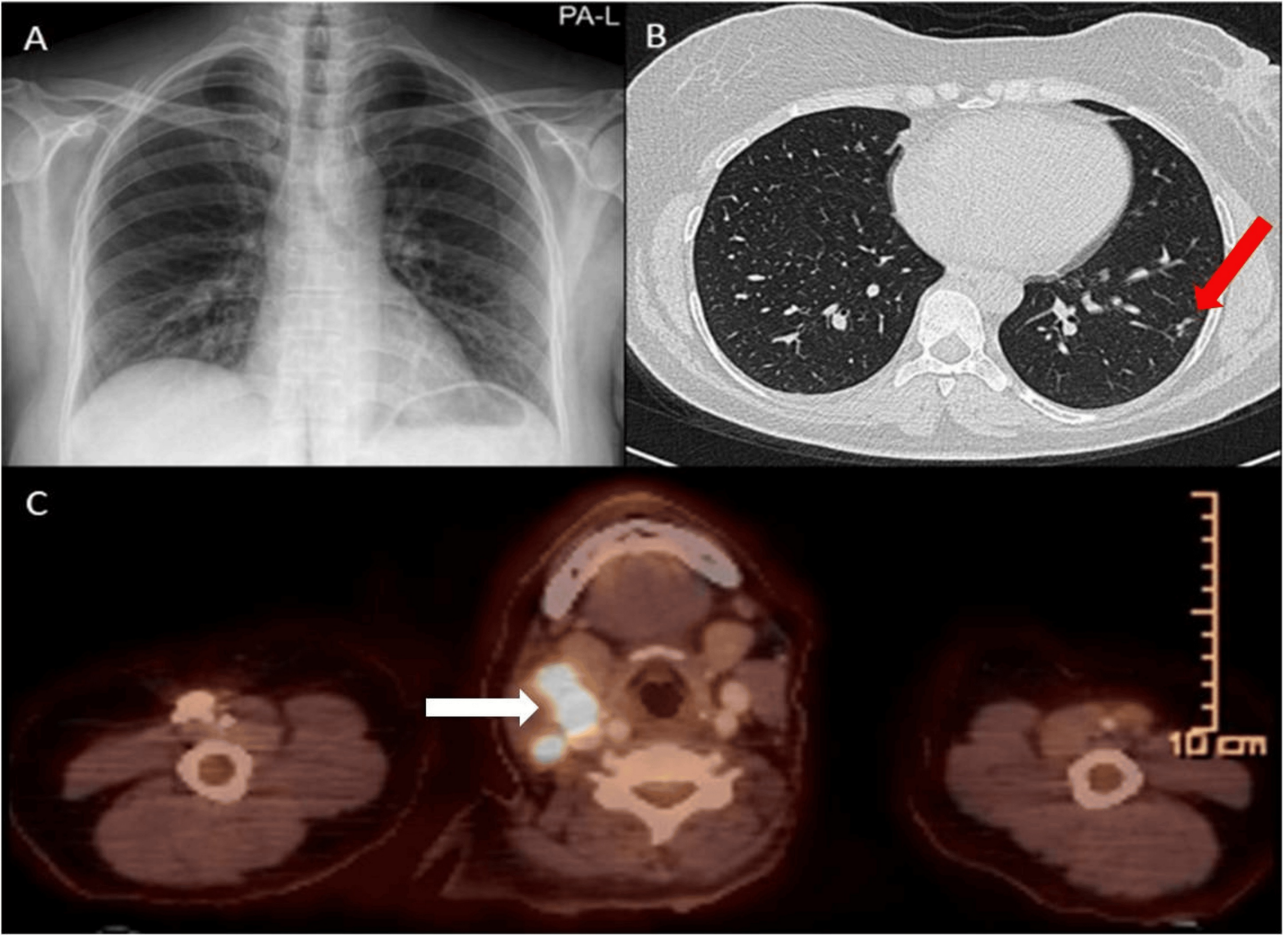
Imaging findings in Case 2 (A) No pathological area observed in chest X-ray; (B) HRCT shows several millimetric nodules in the peripheral area (red arrow); (C) PET/CT shows multiple hypermetabolic lymph nodes; most notably 16 mm (SUV_max_: 14.27) in the right level IIa (white arrow). HRCT: high-resolution computed tomography; SUV_max_: maximum standardized uptake value
